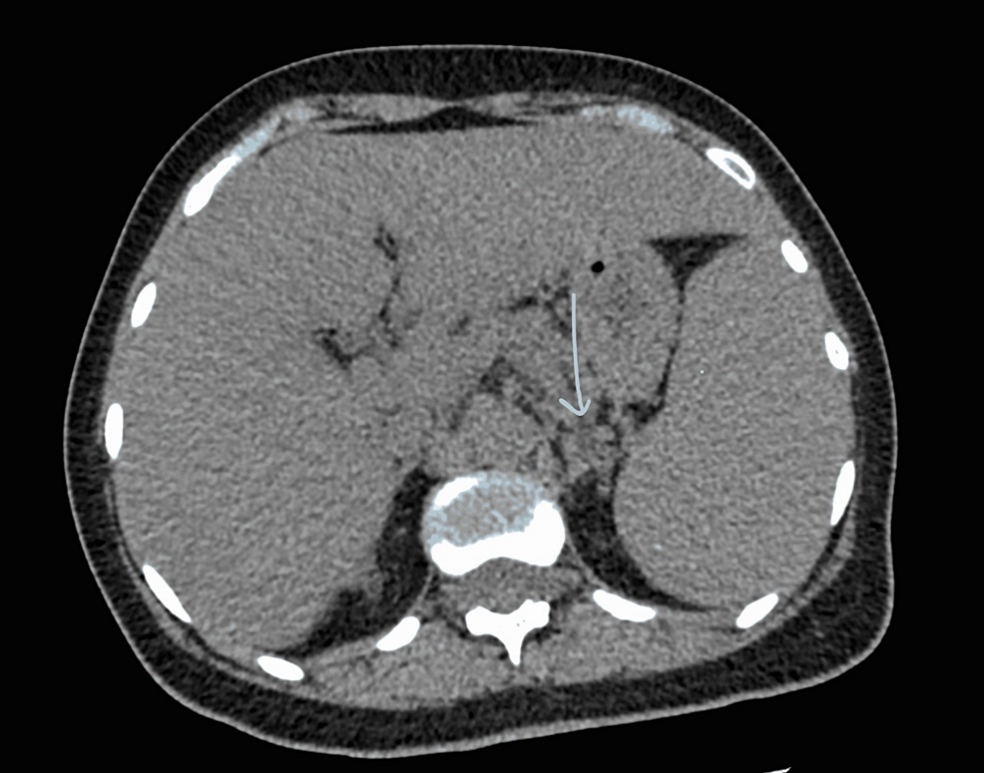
CT scan of the abdomen shows hepatosplenomegaly and abdominal lymphadenopathy
